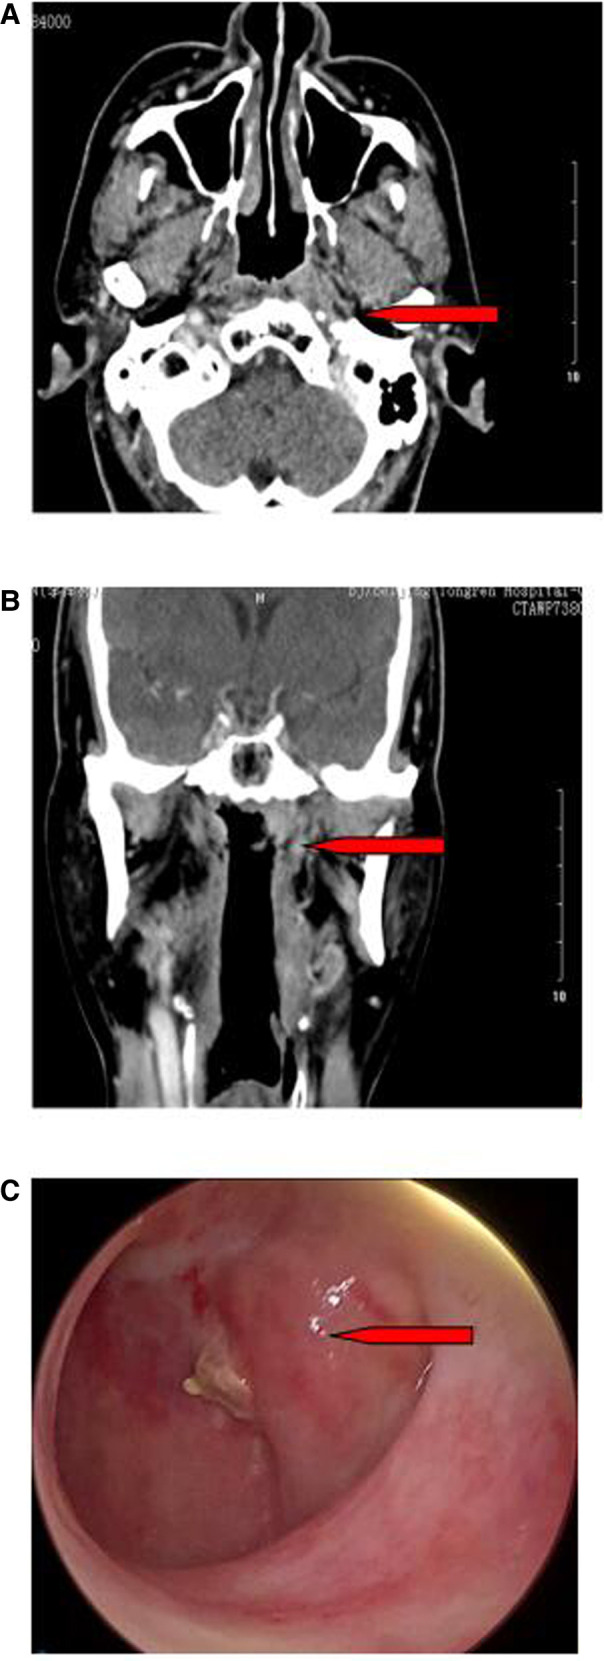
Picture and CT images for the nasopharyngeal tumor (arrows). (A) Axial CT Image. (B) Coronal CT. (C) Picture under nasal endoscopy.
